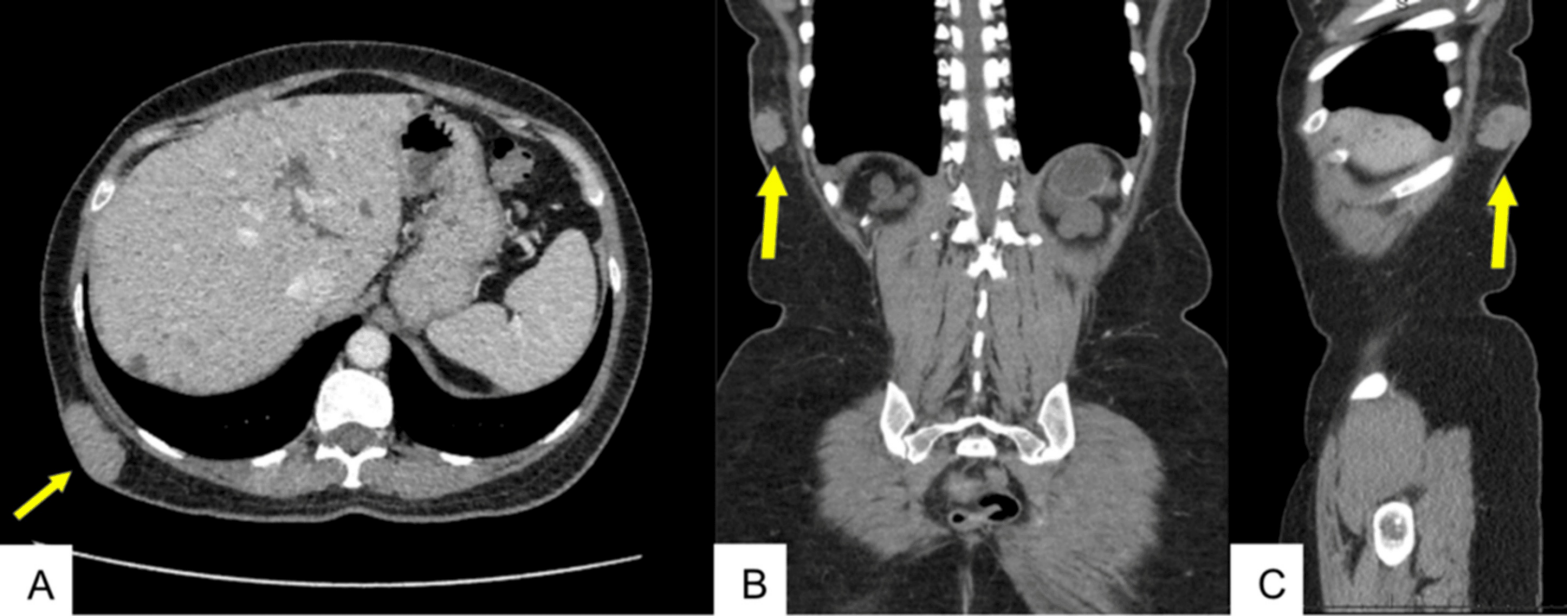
Axial (A), coronal (B), and sagittal (C) CT images from September 2023 demonstrate interval enlargement of the lesion with a more heterogeneous appearance and increased soft tissue volume (yellow arrows), raising concern for a neoplastic process.
